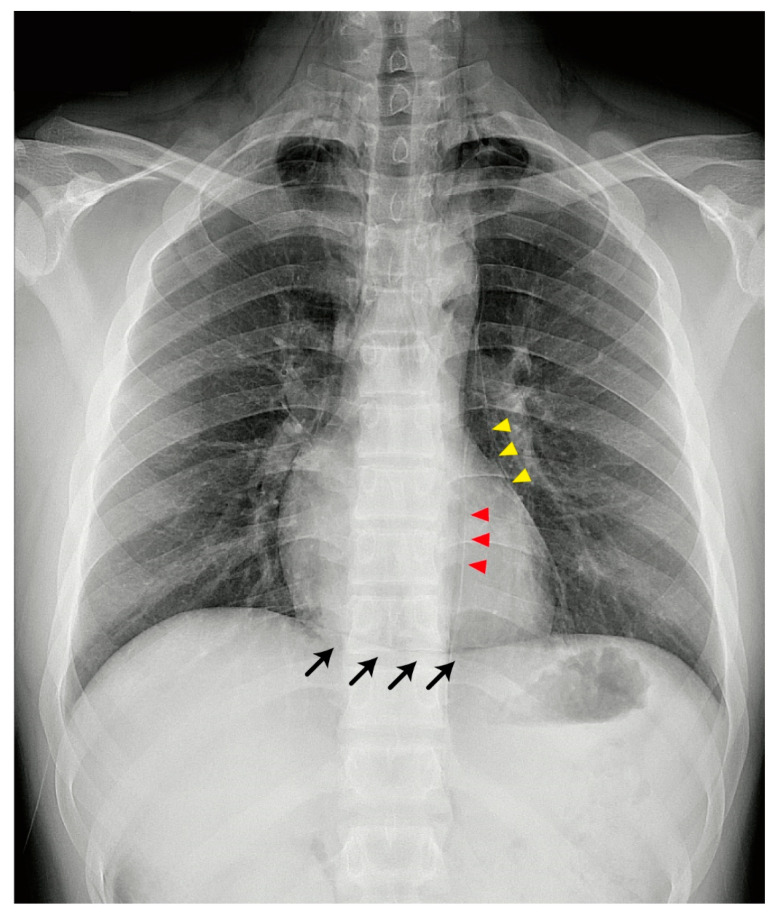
Chest X-ray shows gross subcutaneous emphysema over the neck and pneumomediastinum with a continuous diaphragm sign (black arrows). The gas outlining the mediastinum, aorta (red arrowheads), and heart (yellow arrowheads) extends into the neck.
